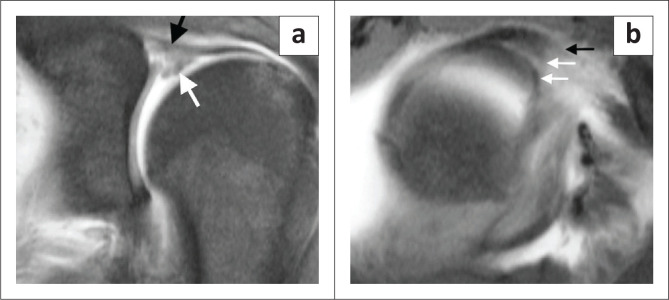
‘Triple structure’ sign. (a) FS T1-weighted (T1W) MR arthrogram coronal image showing inferior displacement of the torn superior labrum (white arrow) and involvement of the biceps anchor (black arrow) of an arthroscopically confirmed SLAP type IV tear. (b) FS T1W MR arthrogram sagittal oblique image demonstrating the ‘three structure’ sign. Black arrow depicts the biceps tendon and white arrows depict the bucket handle fragments.
